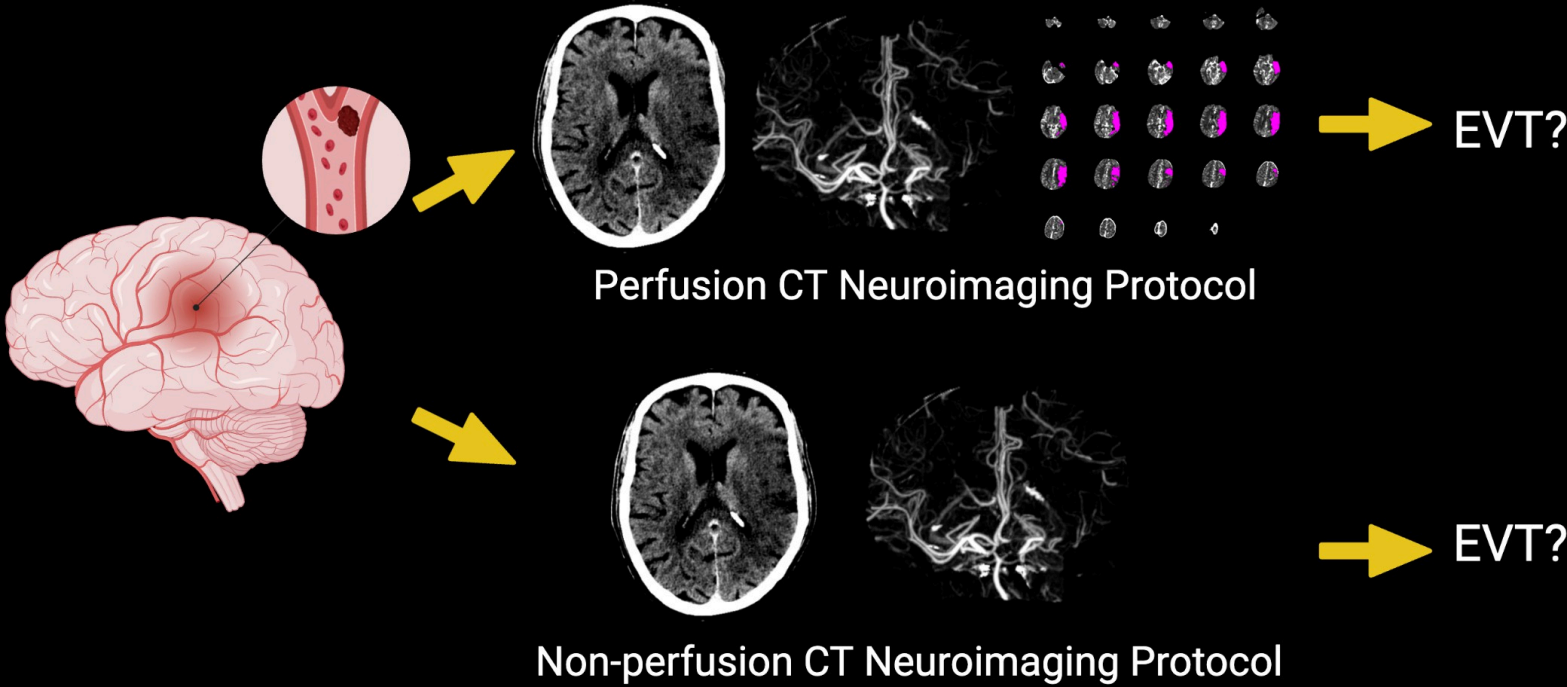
Study protocol. A patient with emergent large vessel ischemic stroke (ELVIS) will be presented to the raters with either of the two neuroimaging protocols: perfusion computed tomography (CT) neuroimaging protocol (non-contrast cranial CT scan (NCCT), computed tomography angiography (CTA), automated perfusion imaging) or non-perfusion CT neuroimaging protocol (NCCT and CTA only). Based on clinical and radiologic details the rater will then decide whether to recommend for or against EVT. (Created with BioRender.com).
